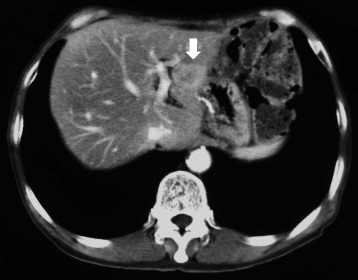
Abdominal computed tomography revealing a 15 mm metastatic lesion in the S2/3 segment of the liver (arrow)
